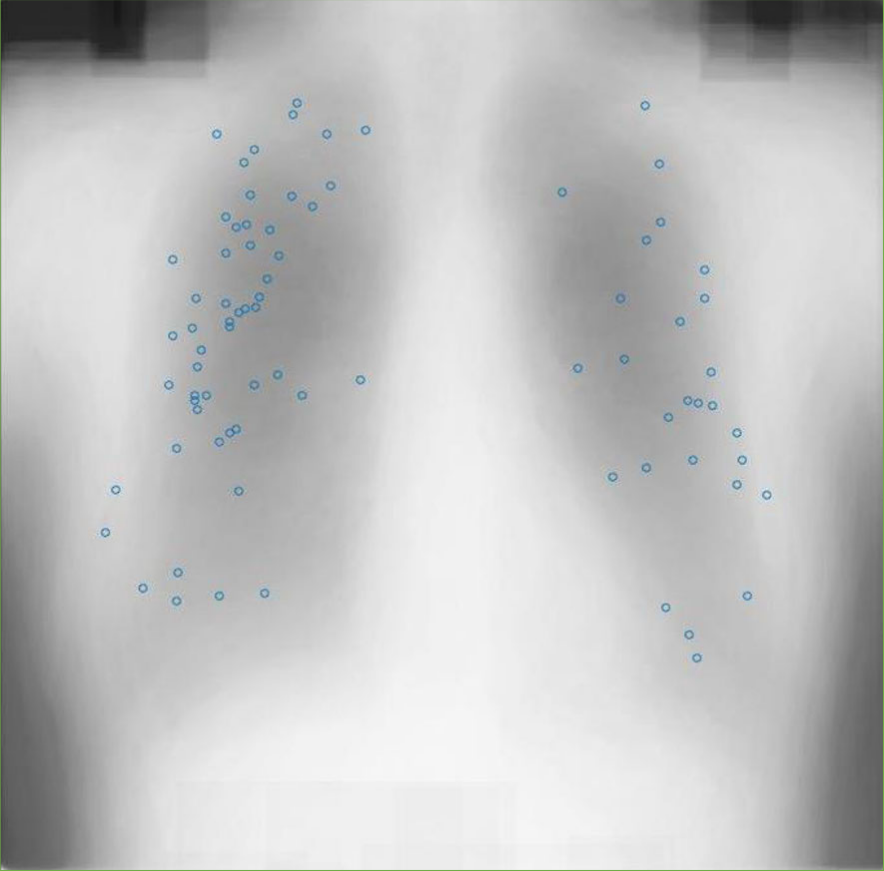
Tumor locations against an average chest radiographic image from the stimuli used in the current study. Each blue dot represents the center location of a tumor from one image
